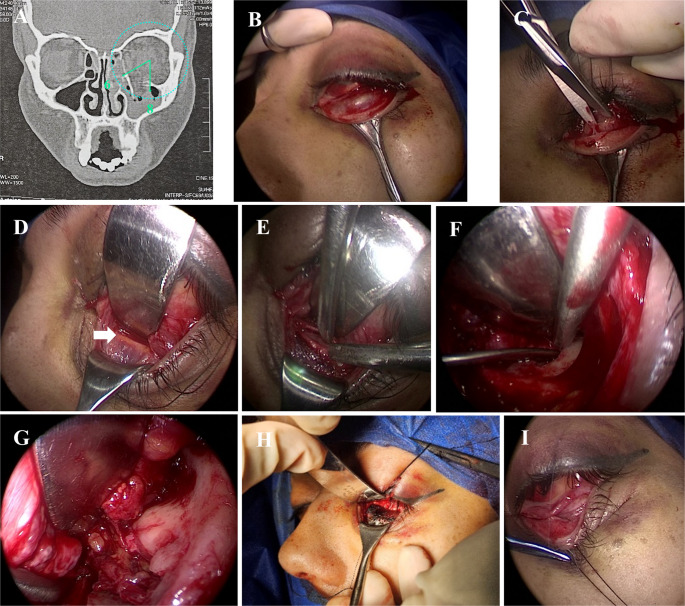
Surgical steps of lower eyelid presetal approach for the inferior orbital portal in case of orbital floor fracture with entrapment of the inferior rectus muscle. A) Coronal CT showing inferior orbital wall fracture with entrapped inferior rectus muscle and fat between 6-8 o’clock. B) Conjunctival incision for lower eyelid. C) Preseptal dissection to avoid orbital fat prolapse. D) Inferior orbital rim was encountered (white arrow). E and F) Subperiosteal dissection was done. G) Release of entrapped fat and the inferior rectus muscle. H) Reconstruction of the orbital floor using titanium mesh. I) Closure of conjunctival incision
